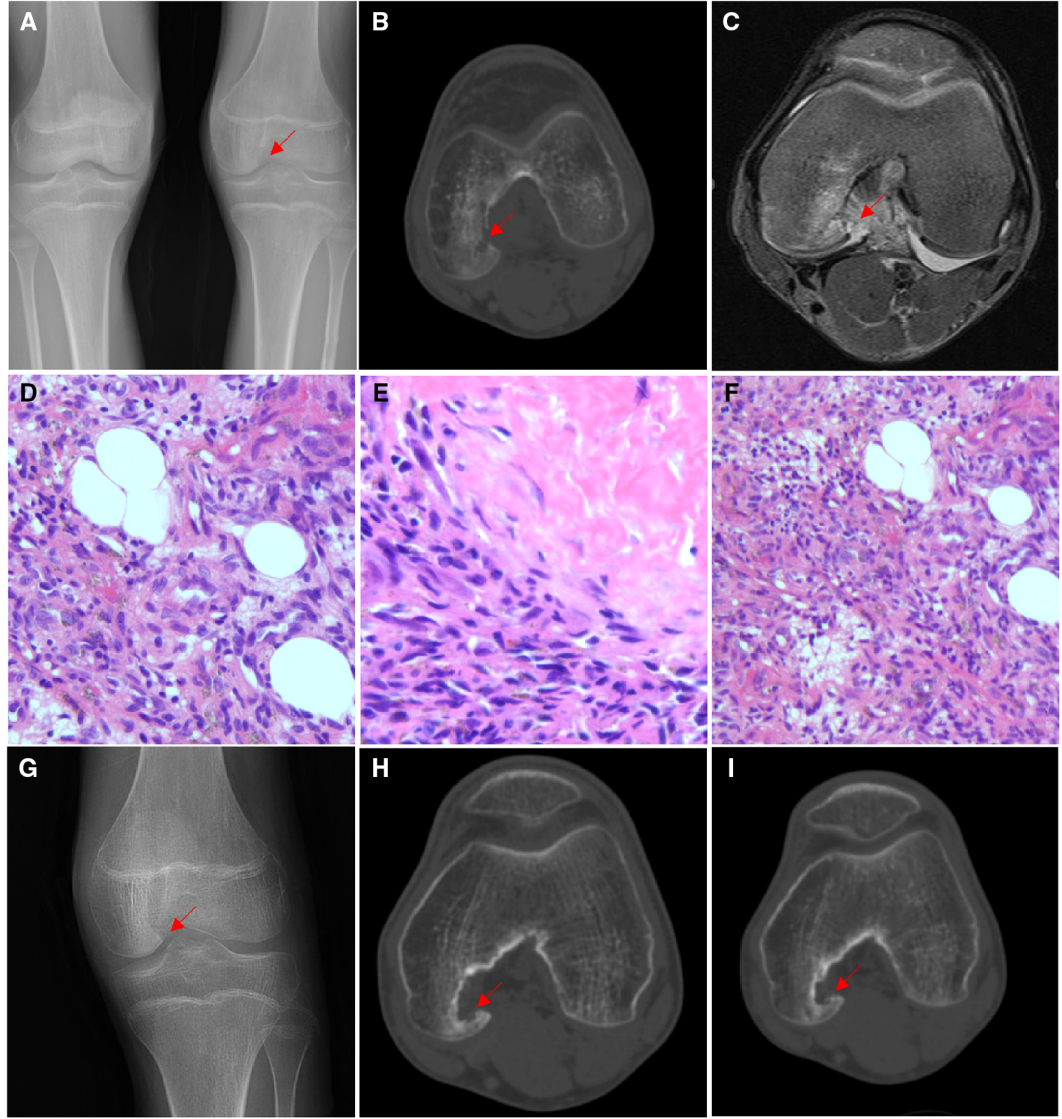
Imaging and pathological features of Case 1. (A) X-ray showed bone destruction of the left medial femoral condyle. (B) CT showed irregular strips of reduced bone density on the medial side of the left femur that were approximately 1.8 cm*0.5 cm, with increased patchy bone sclerosis around the area and local cortical bone destruction at the inner edge. cMRI showed patchy oedema on the lateral side of the left medial femoral condyle with hyperintensity on T2WI-FS. (D) Tumour tissue was distributed in a nodular shape under low-magnification endoscopy, and tumour cells were arranged as onion or concentric circles (HE ×100). (E) Mucoid degeneration or hyaline degeneration (HE ×200). (F) Tumour tissue intersperses between the trabeculae and occupies the medullary cavity tissue (HE ×100). (G) Postoperative x-ray showed bone defect at the posterior edge of the left distal femur. (H) CT showed a limited bone defect near the intercondylar fossa of the left femur medial condyle with slight marginal sclerosis 1 year after operation. (I) CT results 2 years after the operation were not different from those in panel H.
